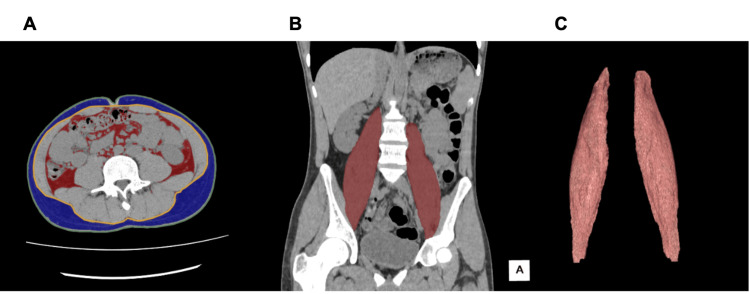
Segmentation and extraction of subcutaneous fat, visceral fat, and psoas major from an axial image obtained with simple abdominal computed tomography. (A) Extraction of visceral and subcutaneous fat. (B) Extraction of the psoas major. (C) Volume rendering image of the psoas major.
